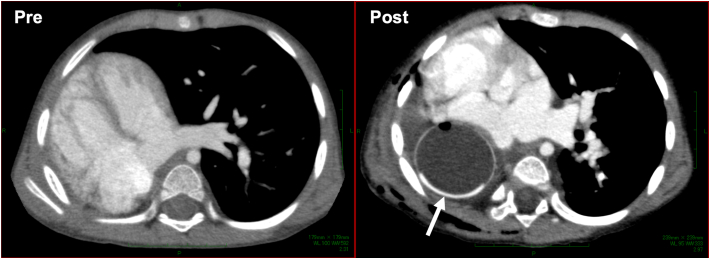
Computed tomography images before (left) and after (right) intrathoracic tissue expander insertion. Arrow indicates the tissue expander.
